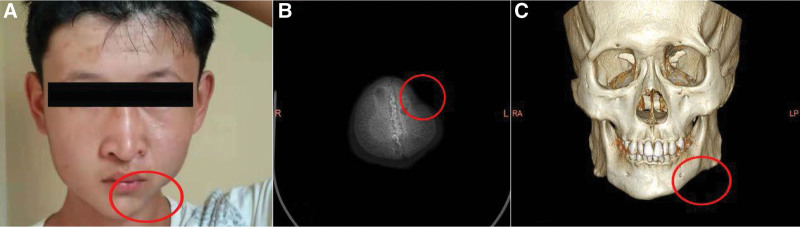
The clinical manifestations of PHA. (A) Frontal view of the patient. (B) A plain CT scan of the patient’s head revealed a narrow bilateral frontal cranial cavity and a significant left cranial depression. (C) A CT scan of the patient’s upper and lower jaw indicated that the left mandible was more concave relative to the contralateral side. PHA = progressive hemifacial atrophy.
